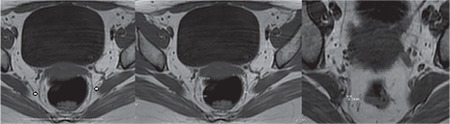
Measurement points of the uterosacral ligament on magnetic resonance imaging
